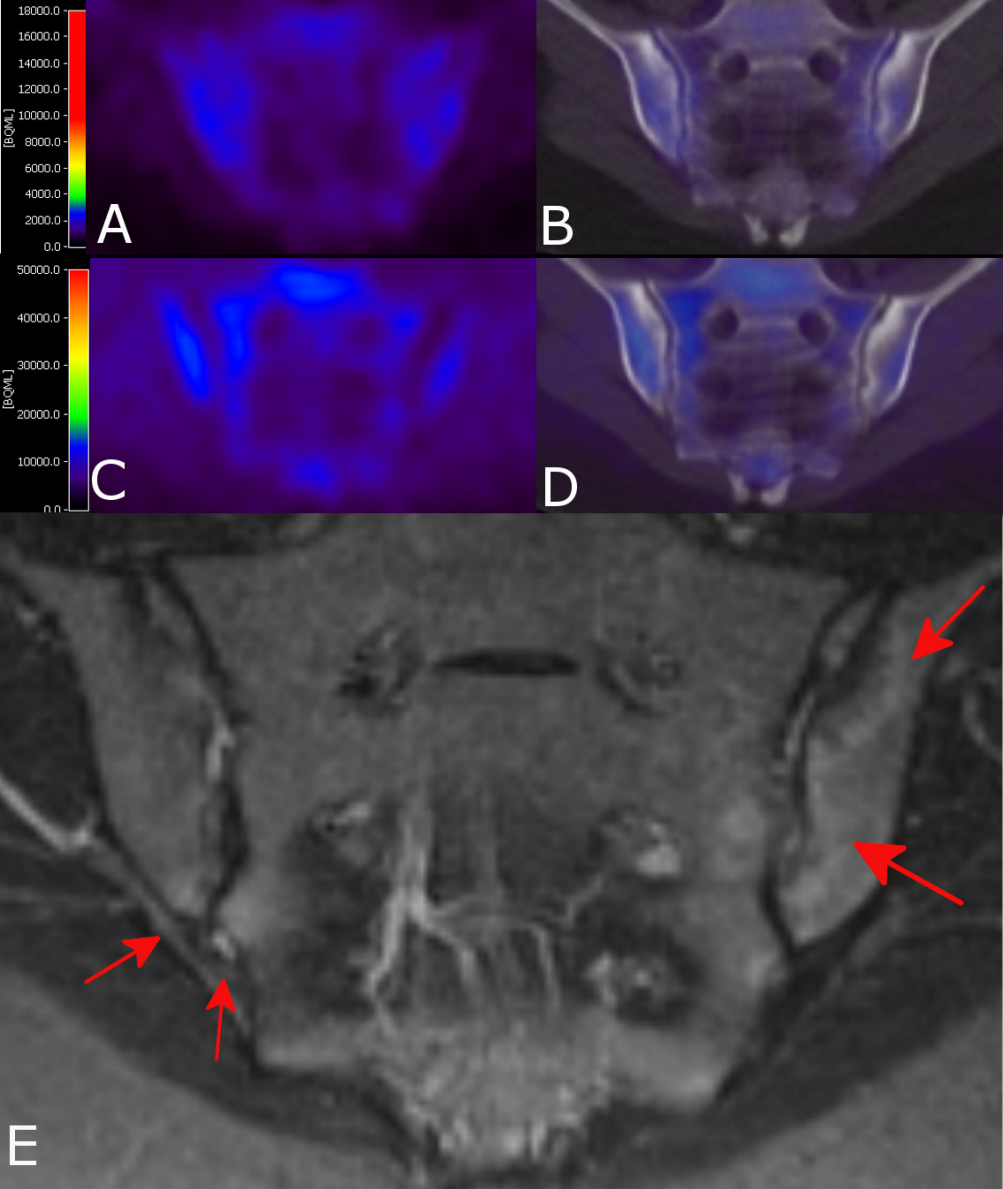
Positron emission tomography (PET) and magnetic resonance imaging (MRI) images of a patient with high disease activity. Coronal [^18^F]FDG (a) PET and (b) PET- computed tomography (PET-CT) and coronal [^11^C](*R*)PK11195 (c) PET and (d) PET-CT images of sacroiliac joints with no tracer uptake. (e) Coronal/oblique MRI (short-tau inversion recovery) of sacroiliac joints of the same patient. Bone marrow edema is present in both sacroiliac joints (indicated by red arrows). [^11^C](*R*)PK11195, PK11195 [(*R*)-1-(2-chlorophenyl)-N-methyl-N(1-methyl-propyl)-3-soquinoline carboxamide]; [^18^F]FDG, [^18^F]-fluoro-2-deoxy-D-glucose.
